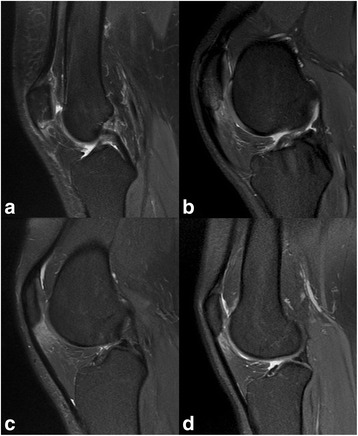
Specific MRI findings in chronic PT: a BME of the inferior patellar pole, b thickening of proximal patellar tendon, c/d IFP edema with infrapatellar bursitis
